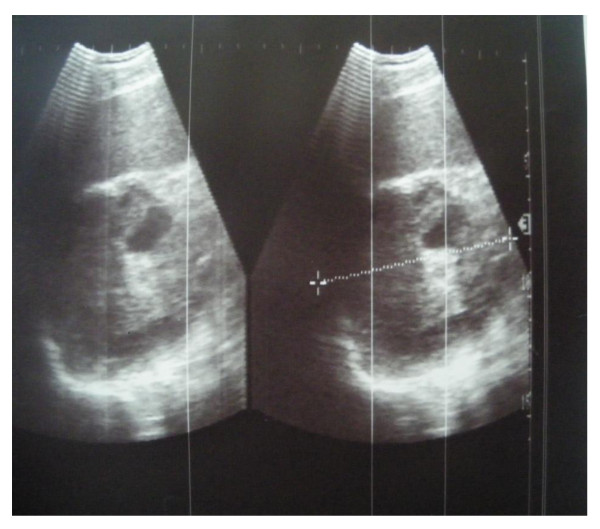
Abdominal ultrasonography revealing a large hypoechoic mass, measuring 10.4 cm in the largest diameter, in the upper pole of his left kidney.
